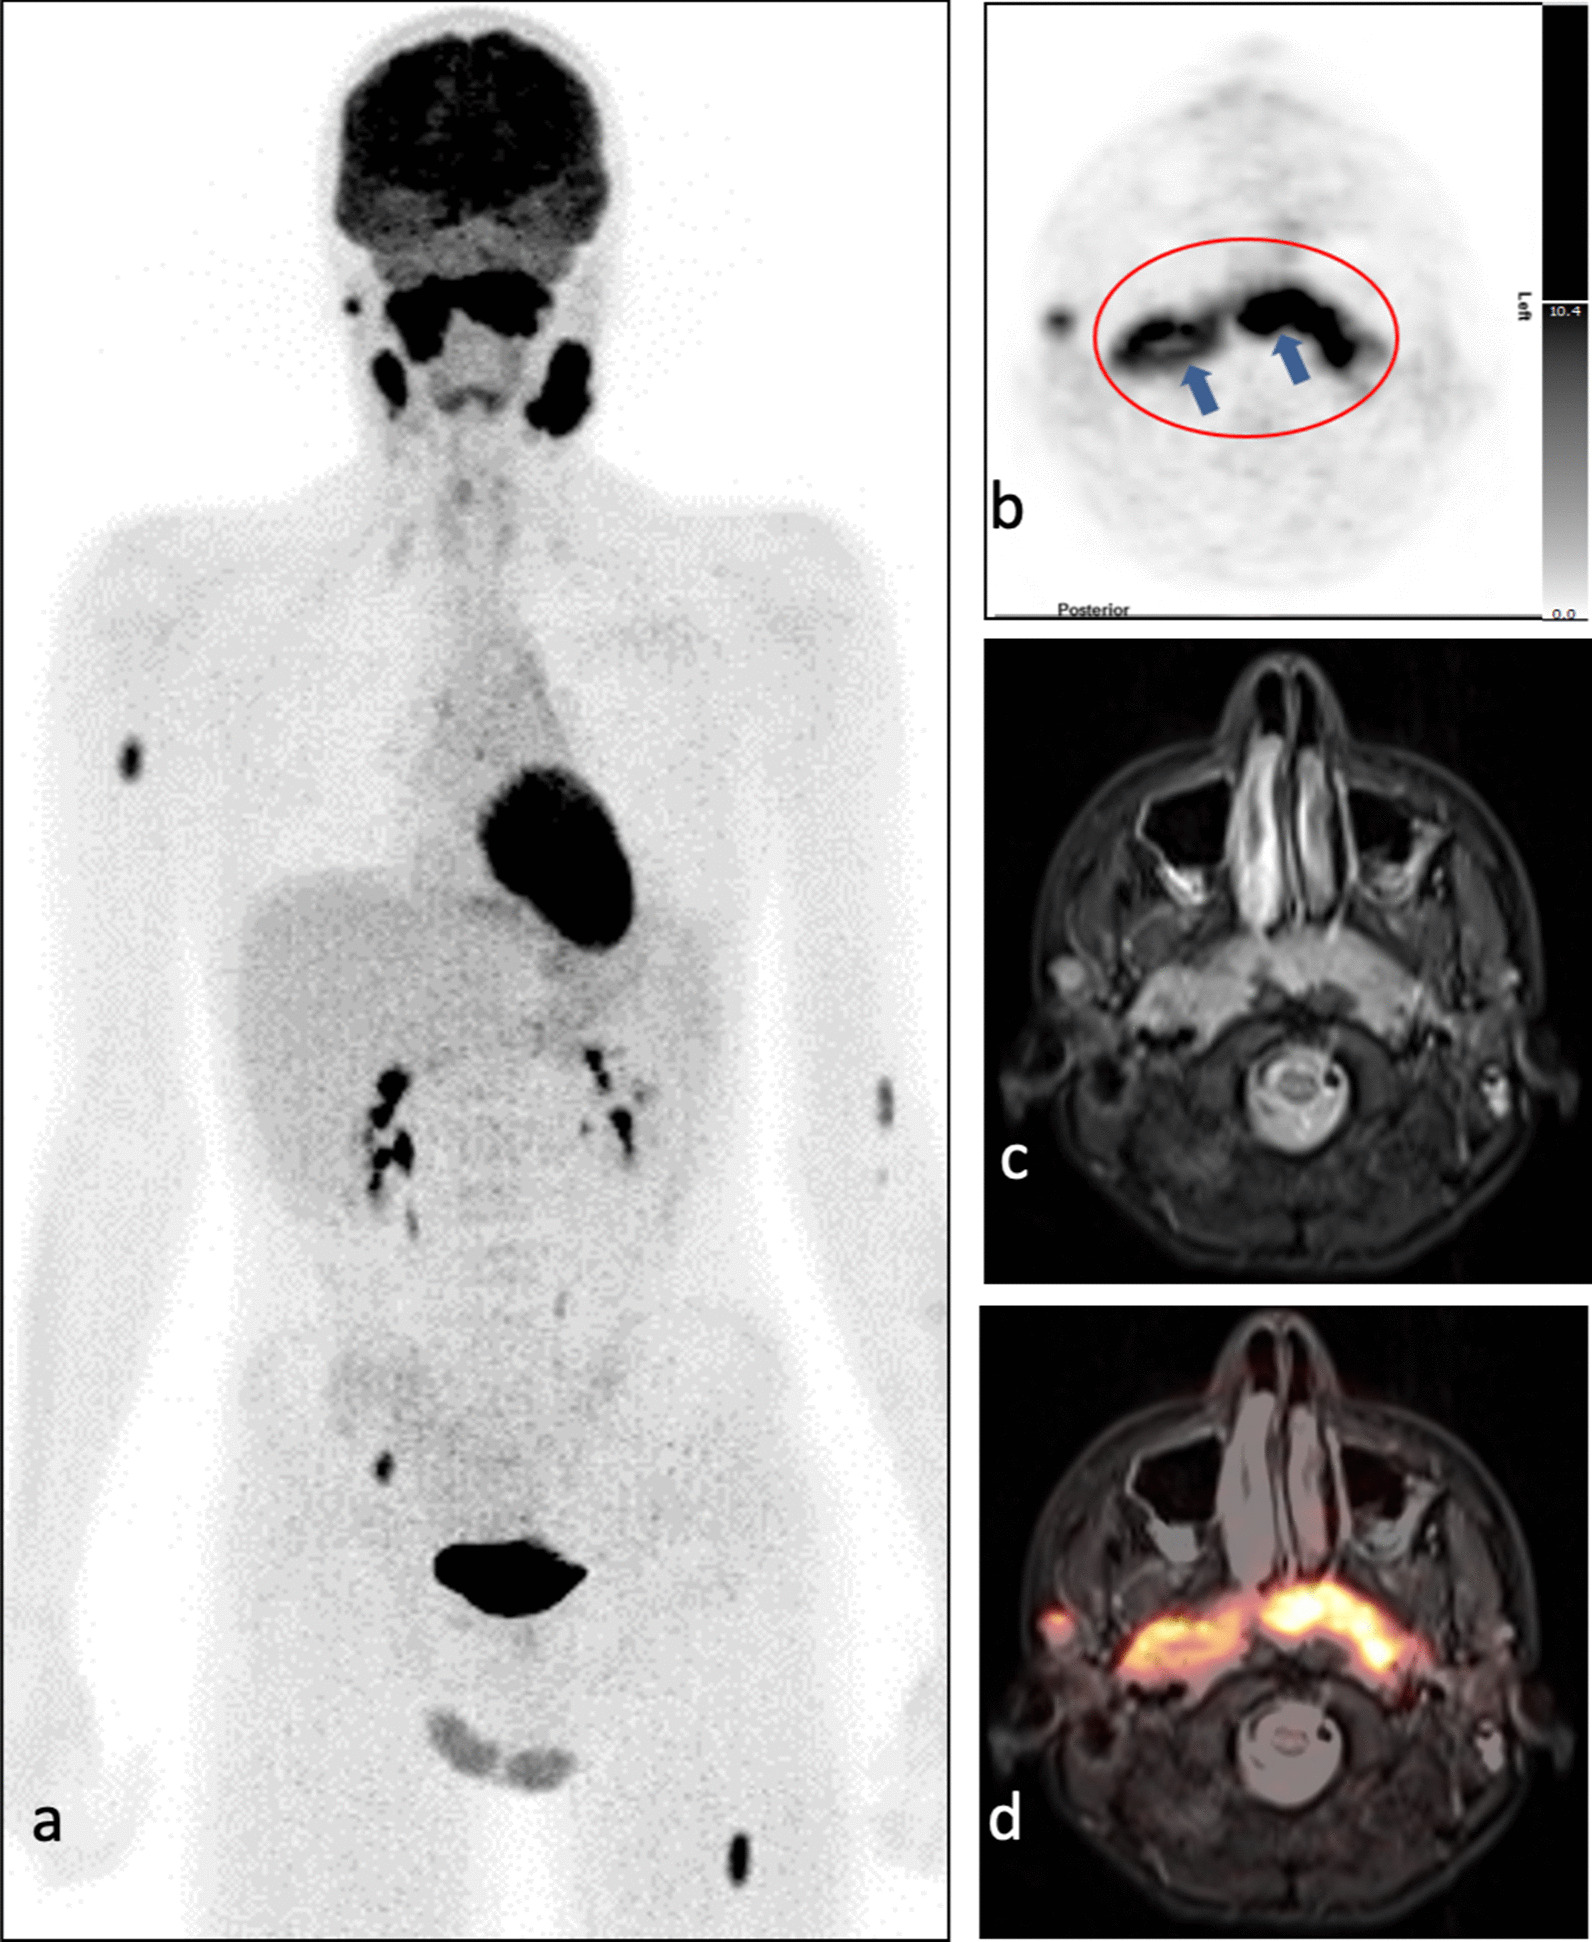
a–d The maximum intensity projection (MIP) shows extensive involvement of the Waldeyer's ring, bilateral cervical lymph node involvement, and three focal lesions in the skeleton (right humerus, right pelvis, left femur) (a). The pharyngeal tonsil (red rim) has asymmetric and inhomogeneous tracer uptake on [^18^F]-FDG-PET images (SUV_max_ right: 14.36; SUV_max_ left: 11.31) (blue arrows) (b, d). On T2-TIRM transversal MR images, the pharyngeal tonsil is markedly enlarged as a result of lymphoma involvement (d)
